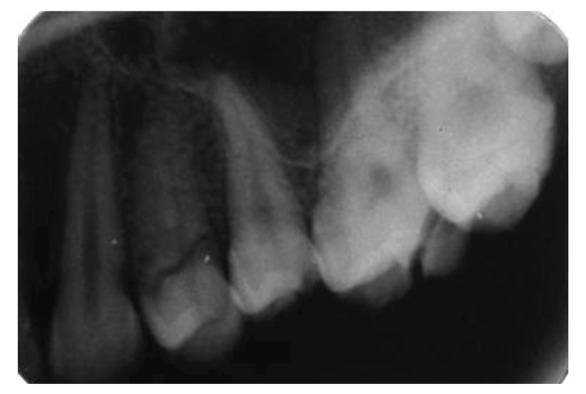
Preoperative radiograph revealing oblique root fracture with 24, loss of buccal cusp with 25, and oblique fracture of distobuccal cusp with 26.
